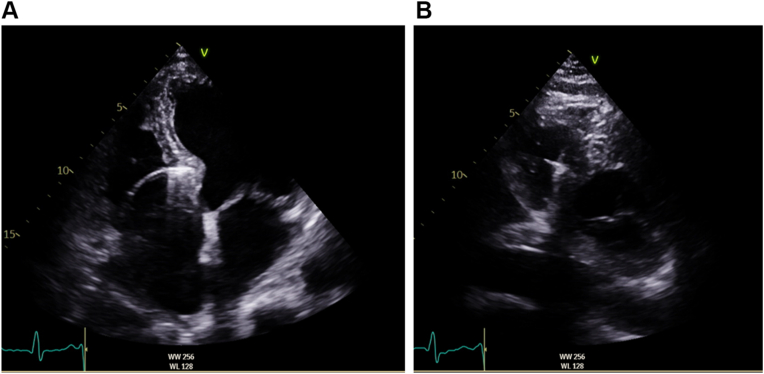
A: Apical 4-chamber view showing the placement of the ventricular lead in the left subendocardial septum immediately after implant. B: Short-axis view showing the placement of the ventricular lead in the left subendocardial septum immediately after implant.
